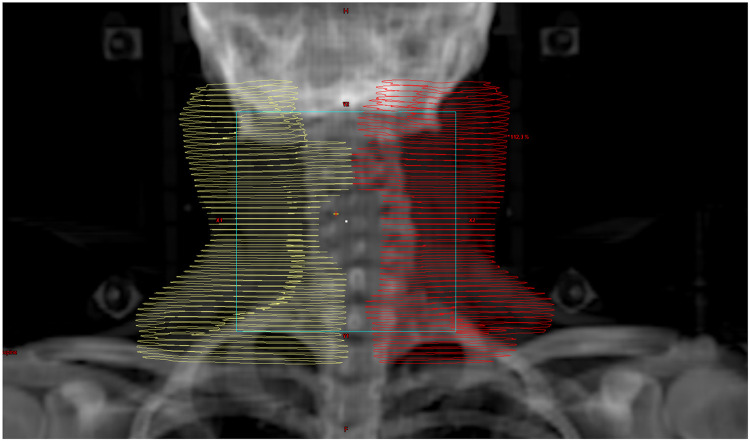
Anterior/posterior digitally reconstructed radiograph demonstrating large bilateral cervical planning target volumes.
